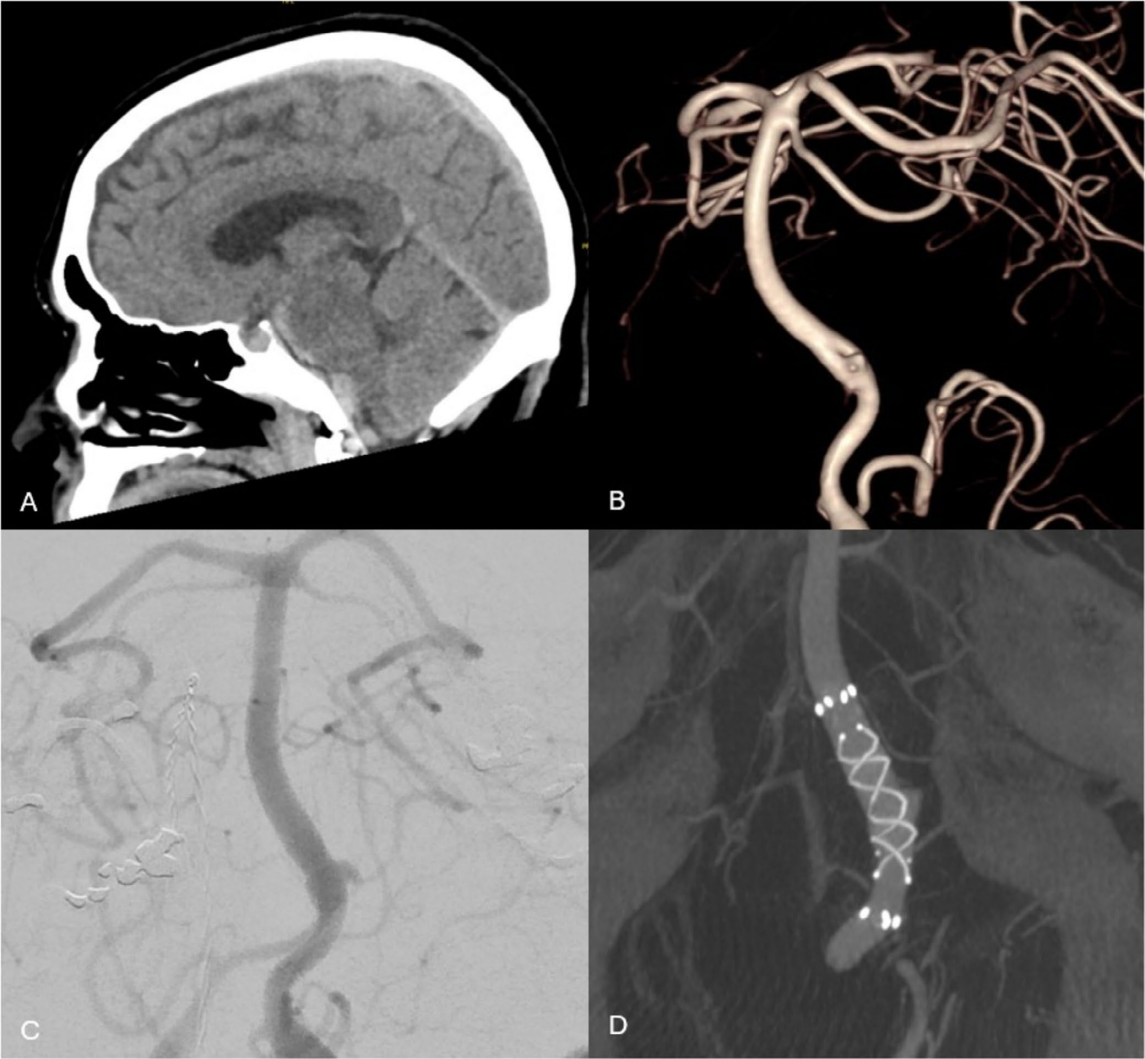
Acute treatment of a 65‐year‐old female with the FRED‐X. The patient presents with WFNS grade 1 subarachnoid haemorrhage with blood in the basal/pontine cisterns and subdural spaces confirmed on CT brain imaging (A). Initial cerebral angiogram was unrevealing. However, a repeat study on day 5 revealed a blister aneurysm at the fenestrated basilar junction (B). AP cerebral angiogram view prior to stenting (C). A FRED‐X flow diverter was deployed across the aneurysm from the basilar artery to the left V4 segment, across the anterior limb of the fenestration. DynaCT shows good wall apposition (D). The patient went on to make an excellent recovery.
